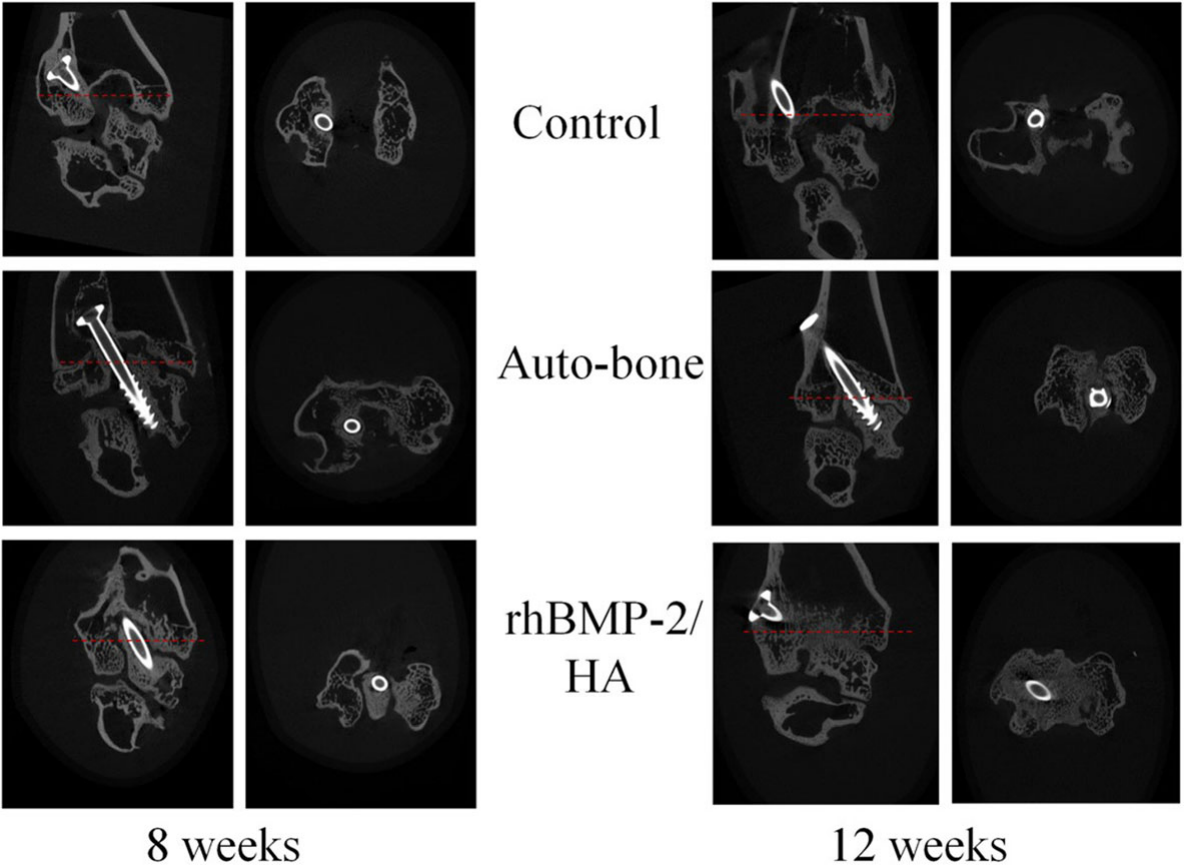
Coronal and transaxial-plane micro-computed tomography images, with red dashed lines indicating the position of the transaxial plane from the coronal plane of the ankle joint at 8 and 12 weeks postoperative
